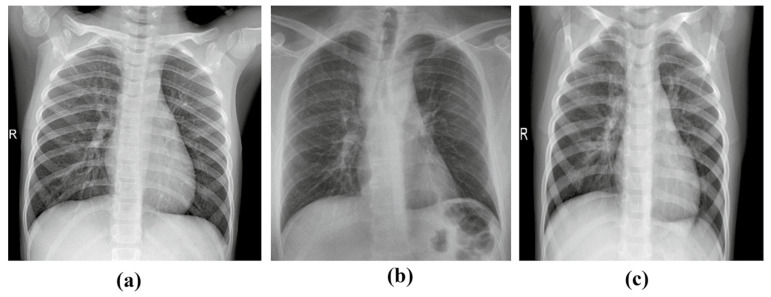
Samples of frontal-view chest X-ray images from the dataset: (a) normal case, (b) COVID-19 case, and (c) pneumonia.
